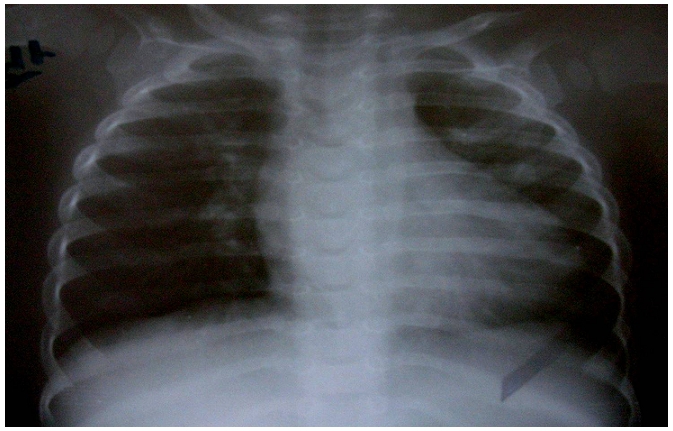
Radiographie thoracique de face; Aspect de cardiomégalie; arc inférieur gauche allongé
